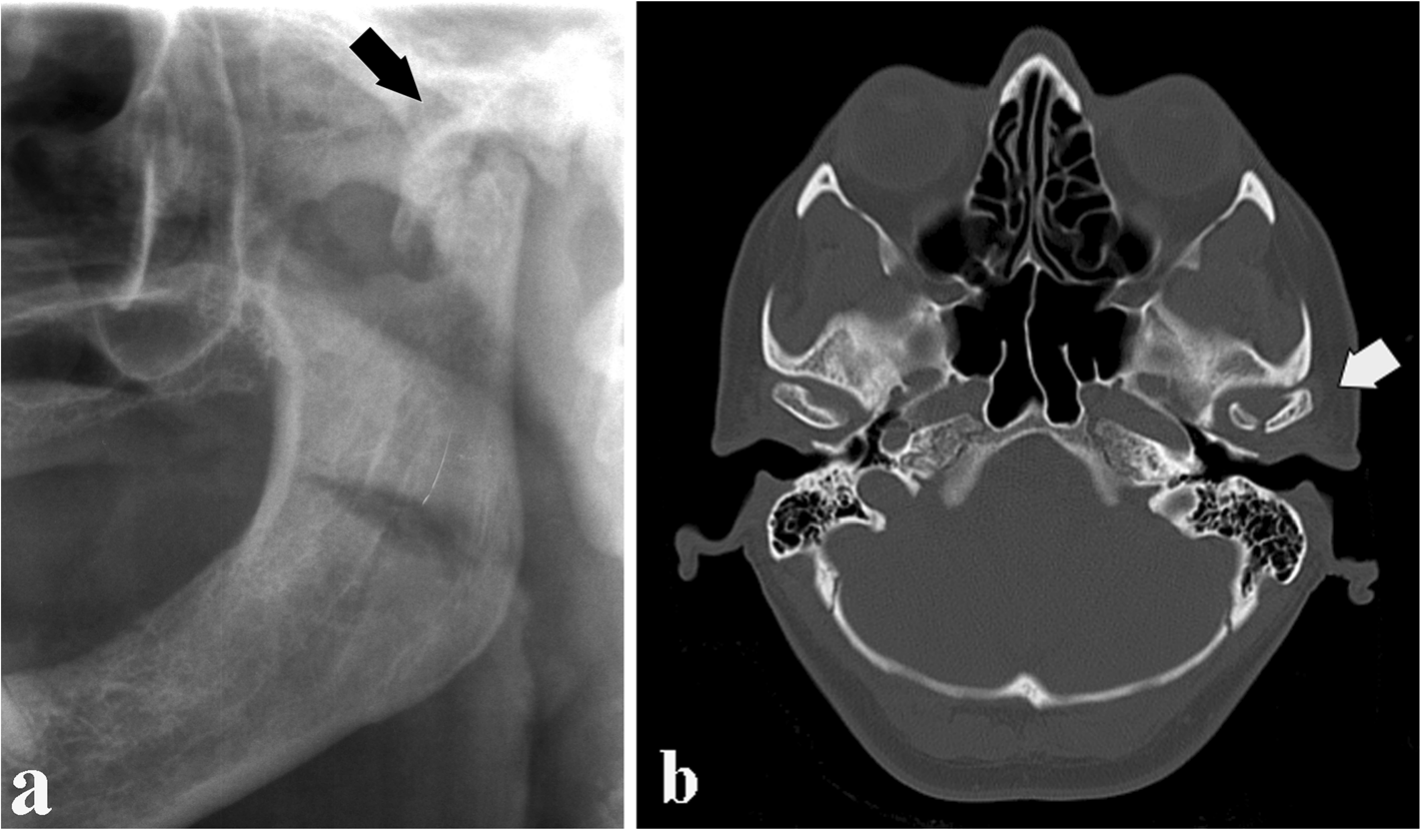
Condylar head fracture. a Cropped panoramic radiograph. b Computed tomography axial section. Oblique fracture of the condylar head with involvement of the articular surface (intracapsular fracture) (arrows)
